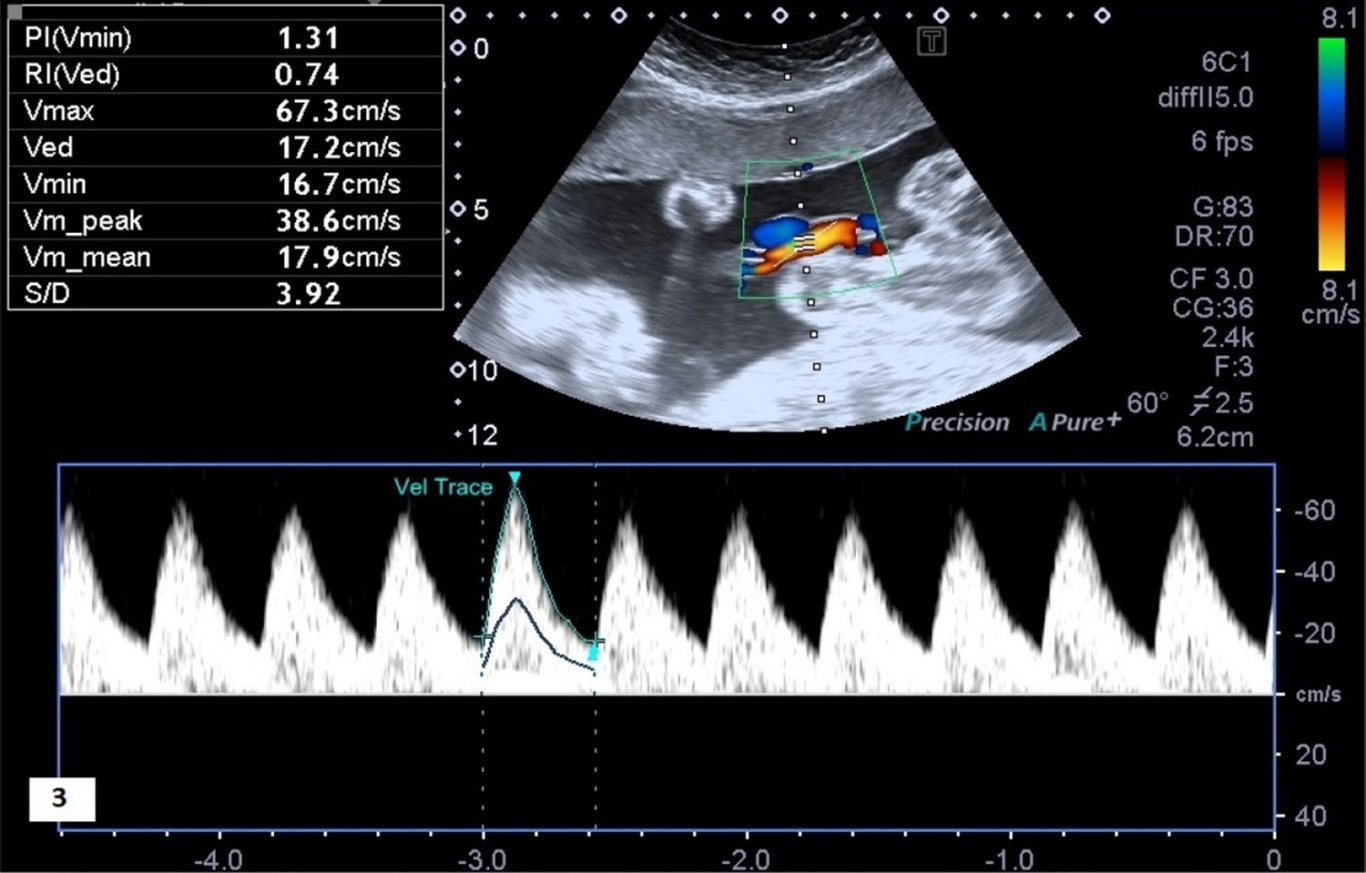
Flow pattern and Doppler ultrasound indexes of the umbilical artery in a three-vessel cord fetus.
